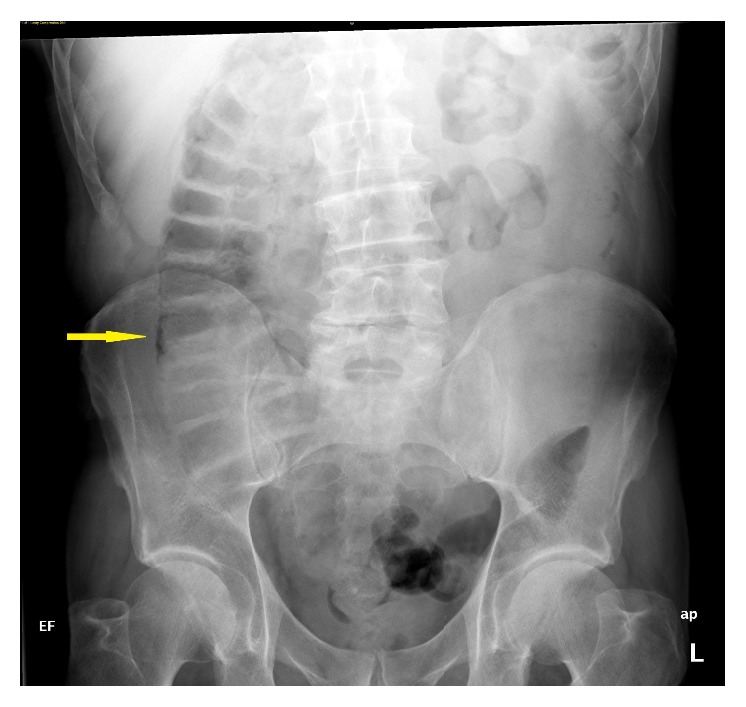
An abdominal X-ray showing pneumatosis intestinalis in the ascending colon of Patient 1.
